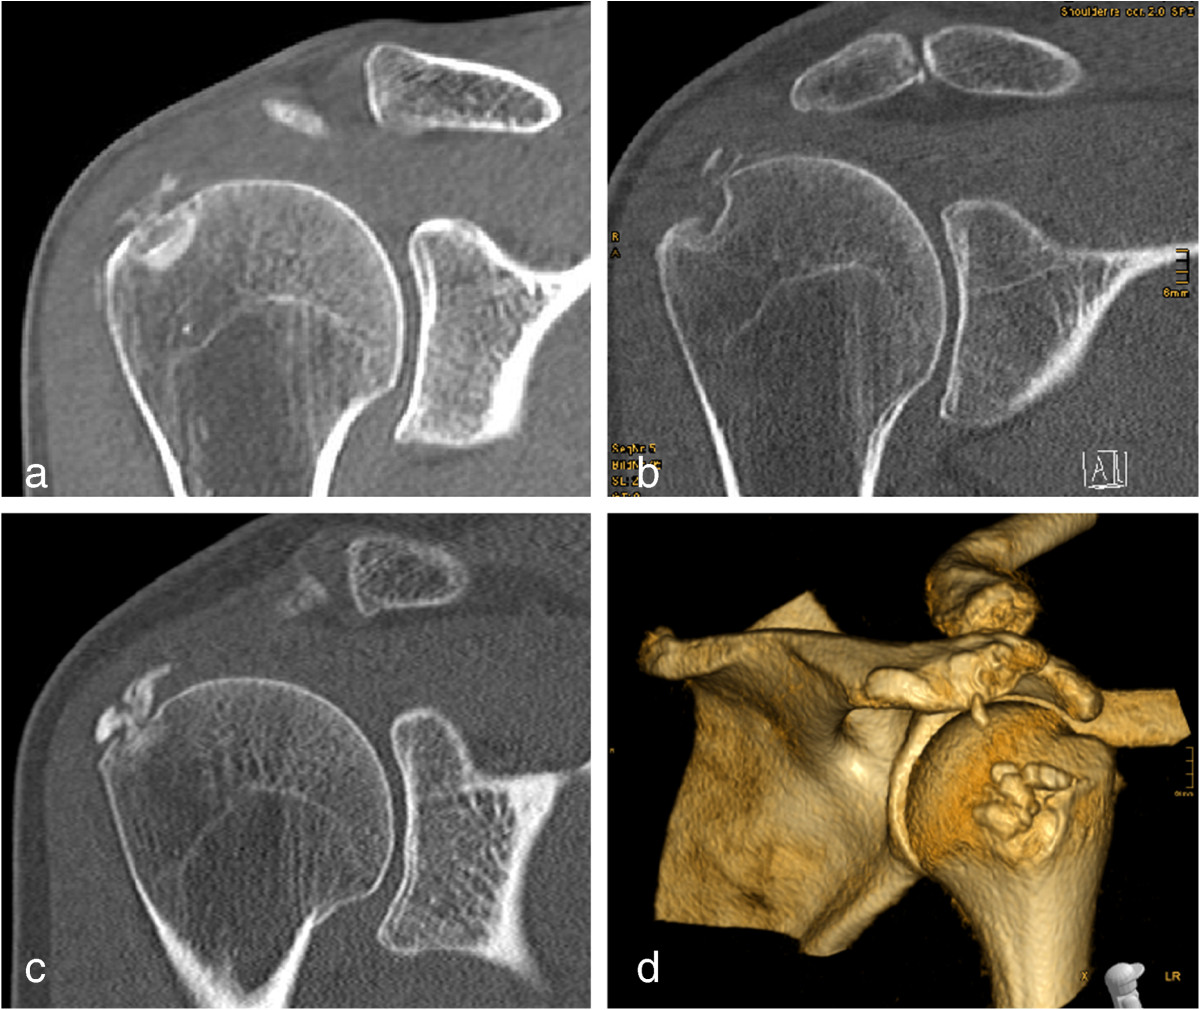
CT scans (a-c) and a 3 D reconstruction (d) showing humeral cysts developing from CD.
